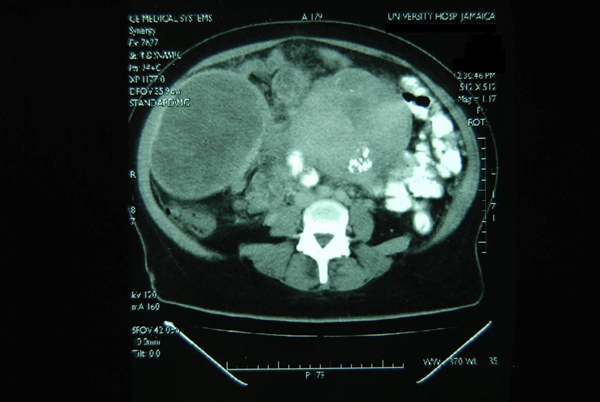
A computed tomography scan showing a large multicystic mass (15.5 × 16 × 9 cm) adherent to the abdominal wall.
